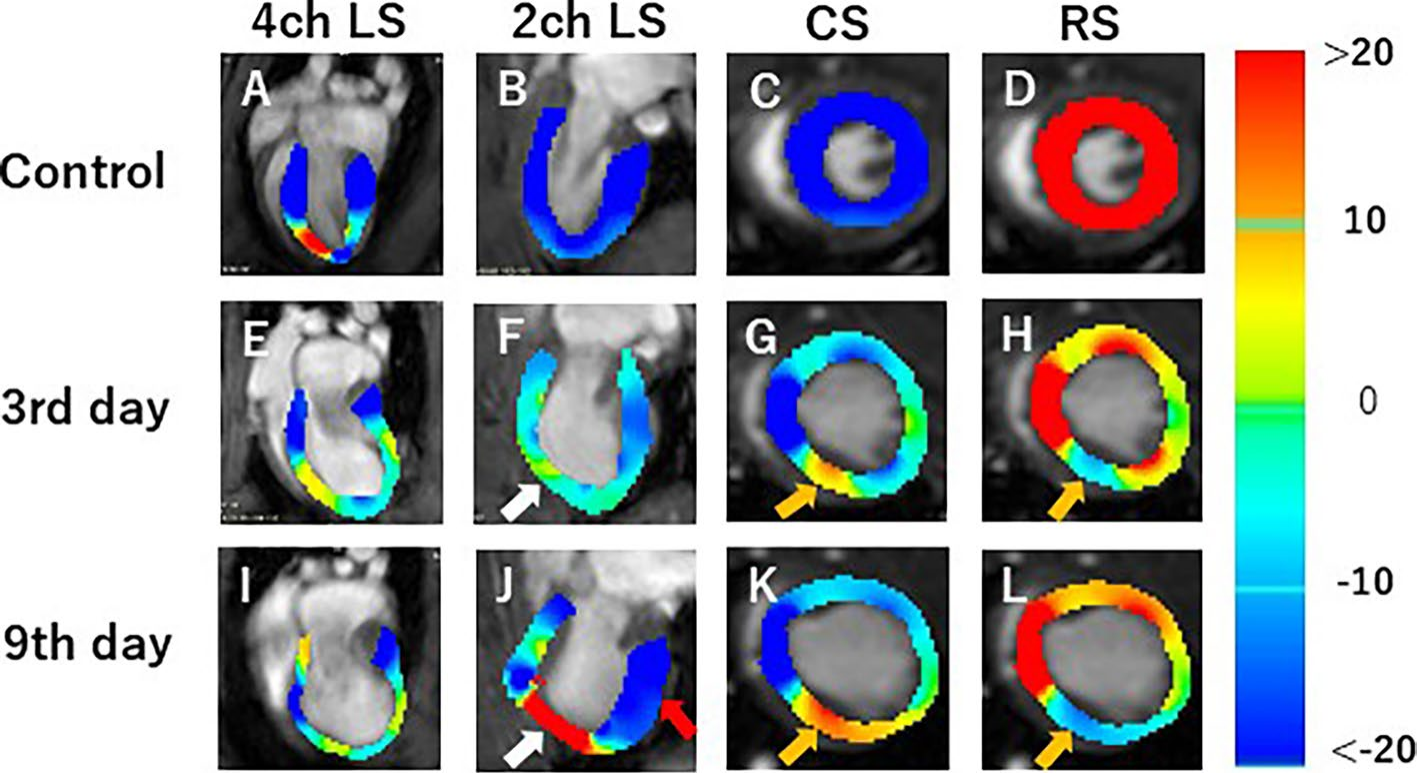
Strain-encoded functional magnetic resonance imaging of the end-systolic left ventricle. 4ch LS, 4ch view longitudinal strain; 2ch LS, 2ch view longitudinal strain; CS, short-axis view circumferential strain; RS, short-axis view radial strain. A, E, I LS in the long-axis 4ch view. B, F, J LS in the long-axis 2ch view. C, G, K CS in the short-axis view. D, H, L RS in the short-axis view. A, B, C, E Control, E, F, G, H 3 days after the onset of myocardial infarction, and I, J, K, L 9 days after the onset of myocardial infarction. The color bar shows the scale of the strain based on the end-diastolic left ventricle, with maximum contraction shown in red and minimum contraction in blue. White arrows: infarcted area. Red arrow: contracting area. Yellow arrows: reduced functionality (cited from Tomography 2023, 9(2), 871–882, Fig. 6)
